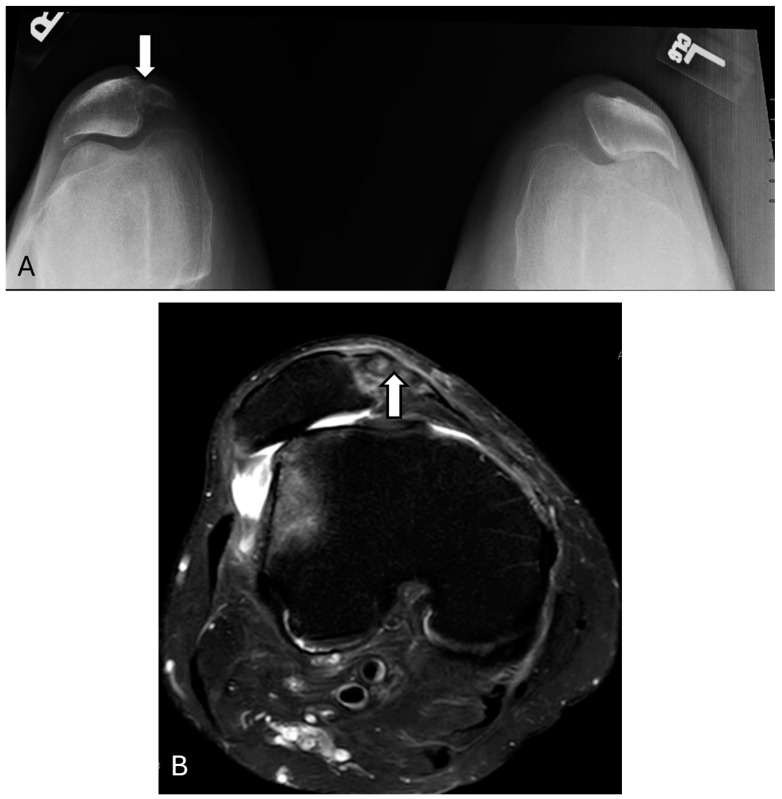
Bipartite patella. The sunrise view knee X-ray (A) shows a right-sided bipartite patella (arrow). Lateral subluxation of the patella, more prominent on the left side, is likely degenerative. Axial proton density image (B) confirms a shallow trochlea with an edematous bipartite patella (arrow) and marrow edema within the lateral femoral condyle. The images were obtained from the University of Washington Medical Imaging database with the required permissions.
